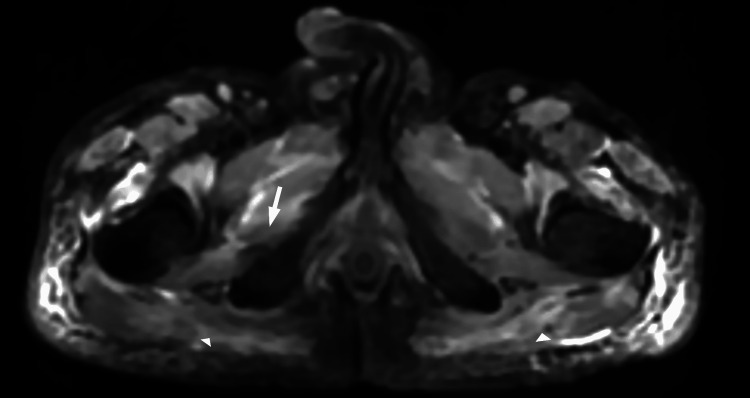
MRI pelvis Inflammation and atrophy of the right obturator externus (arrow) and bilateral gluteus maximus (arrowheads).
